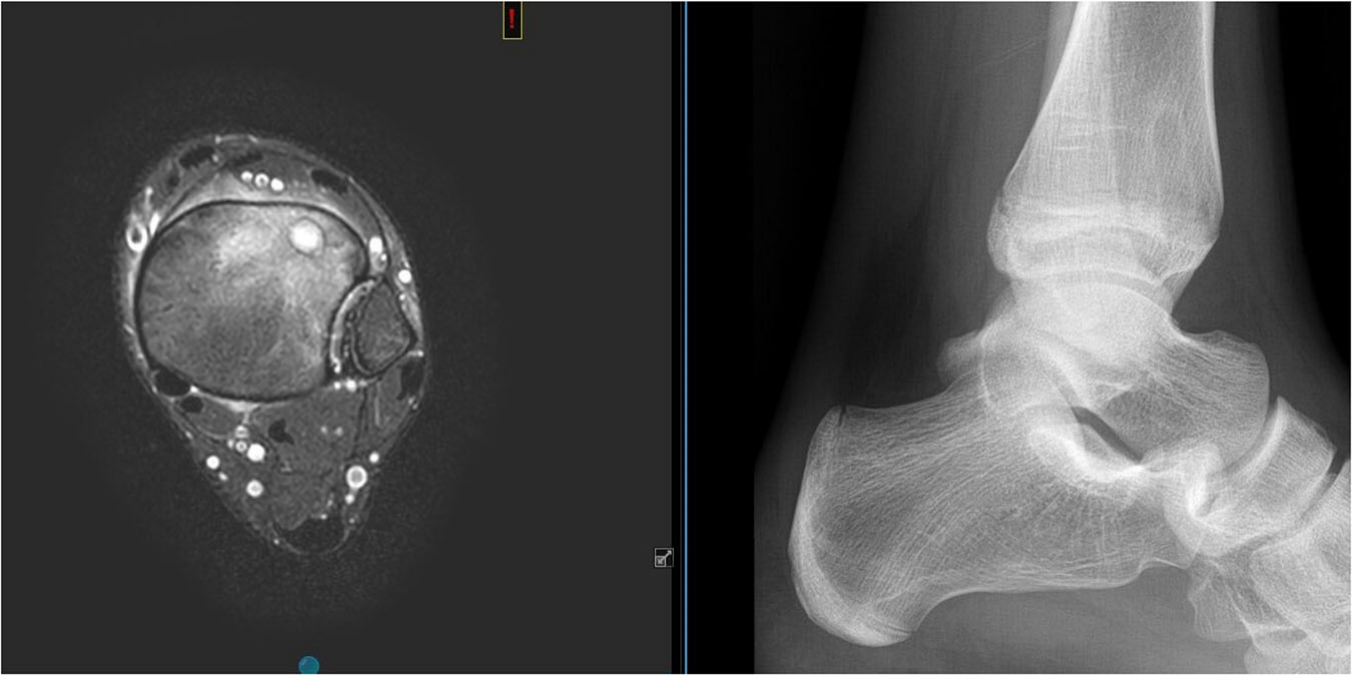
MRI and radiographic view of a Cierny I endomedullary osteomyelitis of the distal tibia (anterolateral). MRI, magnetic resonance imaging [Color figure can be viewed at wileyonlinelibrary.com]
